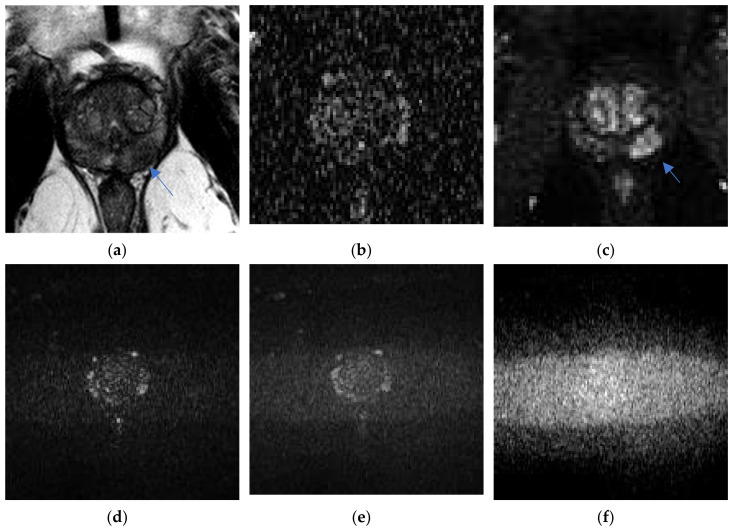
Poor signal to noise with non-endorectal coil (NERC) on high b-value DWI: a 61-year-old man, PSA = 7.8 ng/mL, Gleason = 4 + 3 left mid-gland peripheral zone, cribriform pattern with extra-prostatic extension, only detected (arrow) as T2 hypo-intensity on Philips Achieva 3T NERC T2WI (a) and early enhancement on NERC DCE (c), undetected on NERC ADC (b) and NERC b = 50 s/mm^2^ (d), b = 150 s/mm^2^ (e), and high b-value = 990 s/mm^2^ DWI (f). Images courtesy of University of Chicago.
